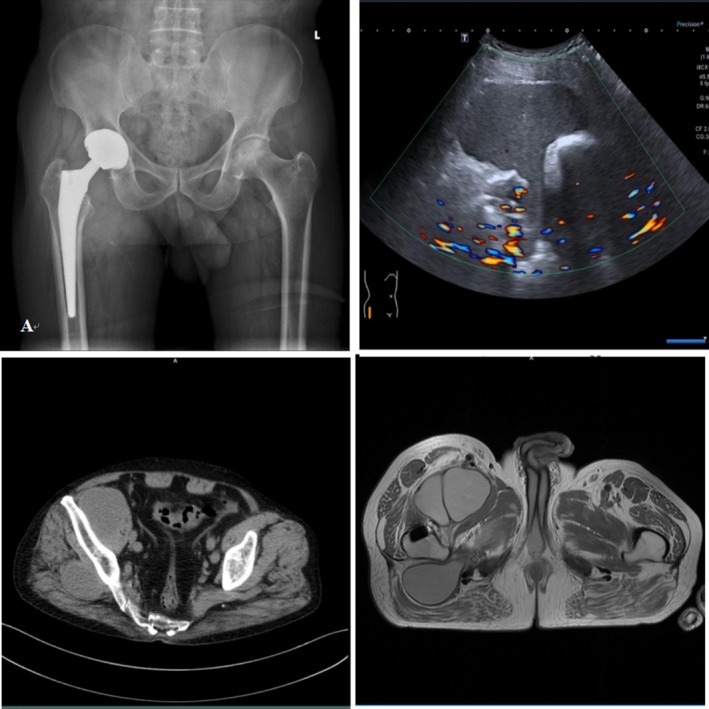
Imaging assessment of IP in a single patient demonstrating the complementary diagnostic value of each modality. The lesion is distinctly visualized on ultrasound, CT, and MRI sequences, whereas the pelvic radiograph obtained in the radiologic department fails to demonstrate the lesion conspicuously. This disparity highlights the limited sensitivity of plain radiography for detecting IP relative to ultrasound, CT, and MRI. (A) Plain pelvic radiograph. (B) Ultrasound with Doppler. (C) Axial CT. (D) Axial MRI.
